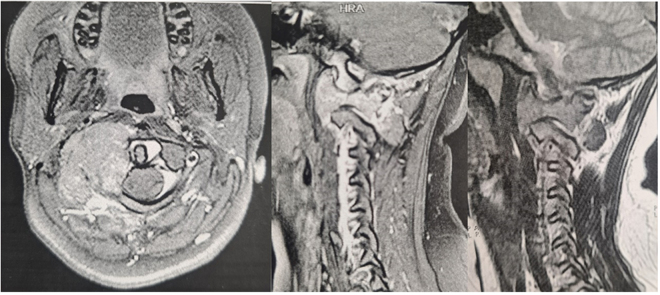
Cervical spine MRI demonstrating a well-defined mass measured 49 × 36 mm in right lateral mass of atlas with encasement of right VA enhancing after gadolinium injection. Left side: lateral view without gadolinium injection; middle: lateral view following gadolinium injection; right: axial view following gadolinium injection.
